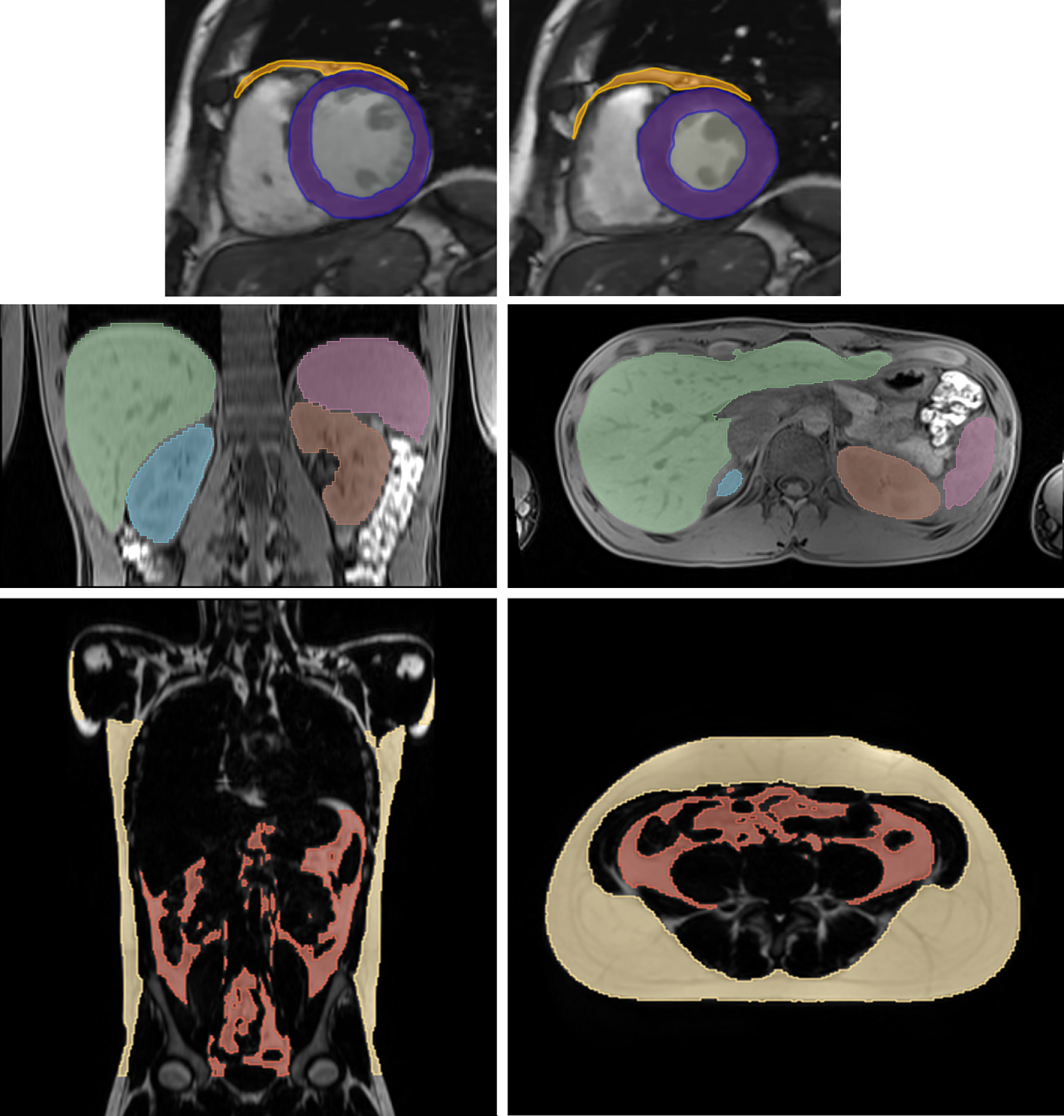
Shows in the top row two short-axis views of the heart in diastole (left) and systole (right). The myocardium of the left ventricle is segmented and coloured purple, areas of epi-/pericardial fat are depicted in orange. The middle row shows MR images with segmentation of internal organs as liver (green), spleen (light purple) and left (brown) and right (blue) kidneys. The two images in the bottom row highlight the subcutaneous fat (yellow) and visceral fat (red)
